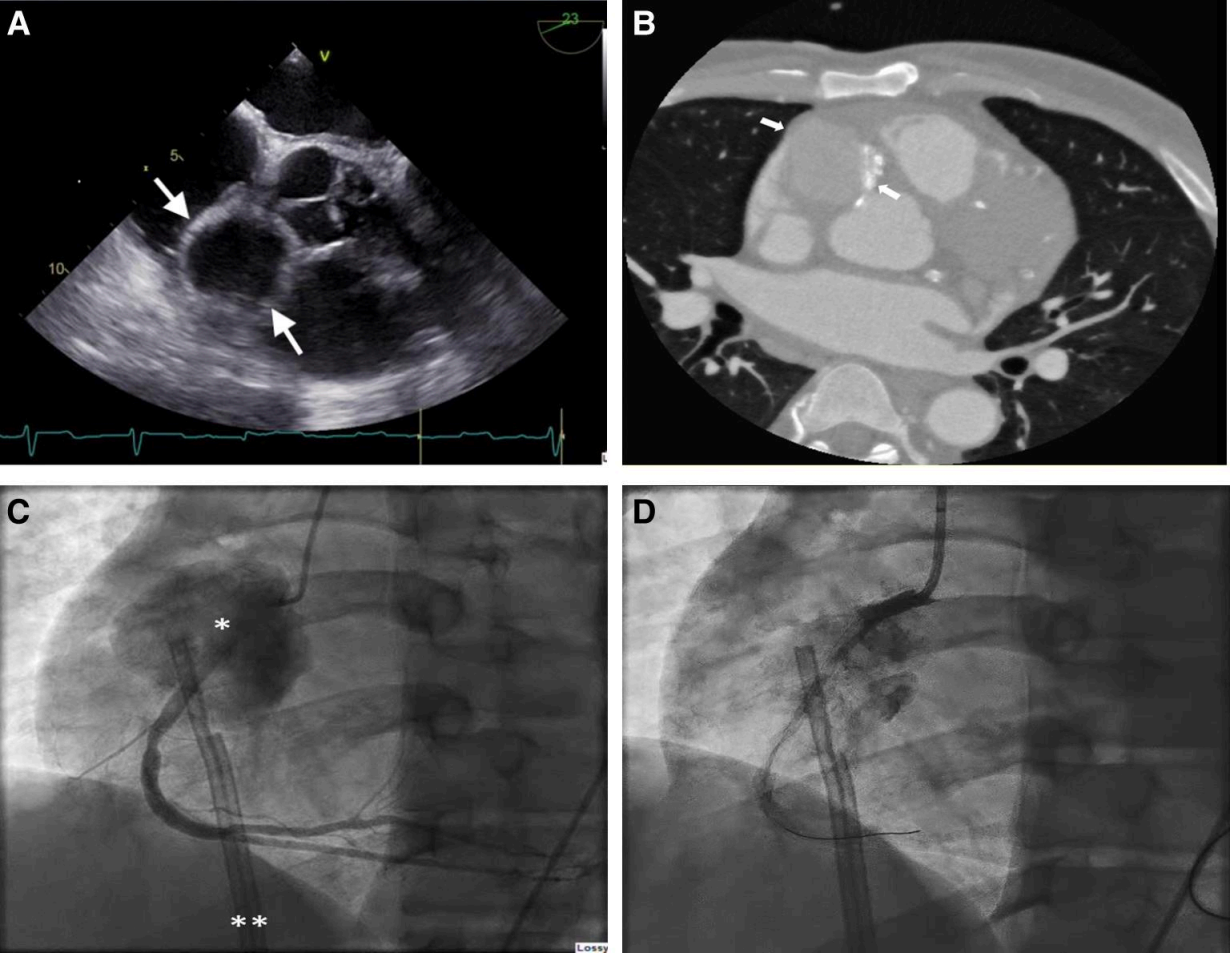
(*A*) Transoesophageal echocardiogram on second presentation showing a large cystic structure (4.0 × 3.5 cm) on mid-oesophageal short-axis view at the aortic valve level, (*B*) computed tomography angiography showing right coronary artery pseudoaneurysm (between the arrows) abutting aortic root; the dense linear radio-opacity on the medial side of the pseudoaneurysm is a drug-eluting stent, (*C*) second presentation angiogram: left anterior oblique view of the right coronary artery showing the pseudoaneurysm labelled ‘*’ and a temporary femoral dialysis catheter labelled ‘’, (*D*) percutaneous intervention of pseudoaneurysm: right coronary artery angiography after covered stent deployment in proximal right coronary artery. Note contrast flow distal to the covered stent into the pseudoaneurysm. DES, drug-eluting stent; RCA, right coronary artery.
